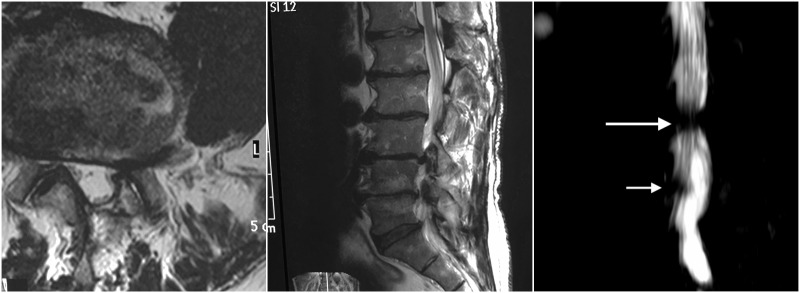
Lumbar spine magnetic resonance imaging (MRI) showing spinal stenosis. Axial MR image at L4-5 on T2-weighted imaging. Subarticular stenosis on right side is observed. Note partial laminar defect on left side, secondary to previous surgery in that area. Left subarticular stenosis is also observed. Center. Right parasagittal MR on T2-weighted imaging. Stenosis is observed at L3-4 and L4-5 segments. Modic changes are present mainly at L4-L5. Right. MR myelography. Bilateral stenosis is observed at L3-4 (large arrow), right stenosis at L4-5 is also demonstrated compressing the right L5 nerve root (small arrow).
